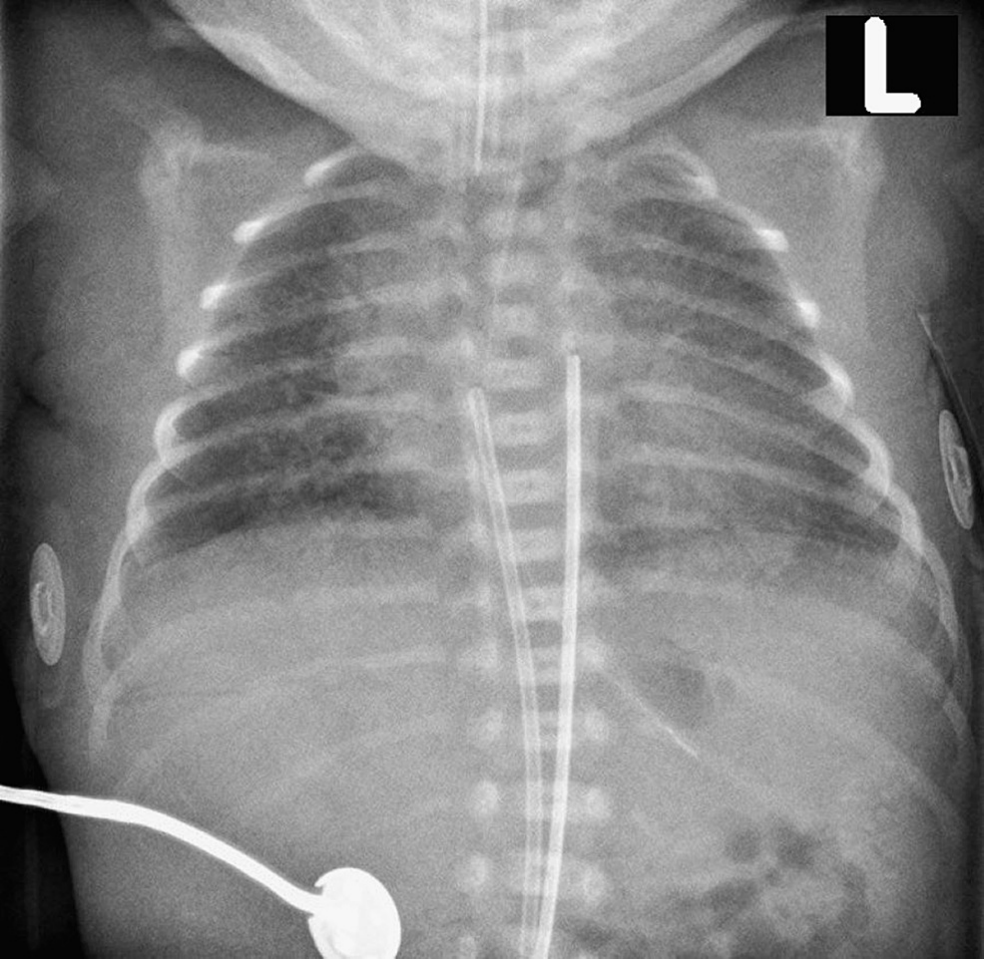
X-ray of the baby showing bilateral perihilar haziness.
